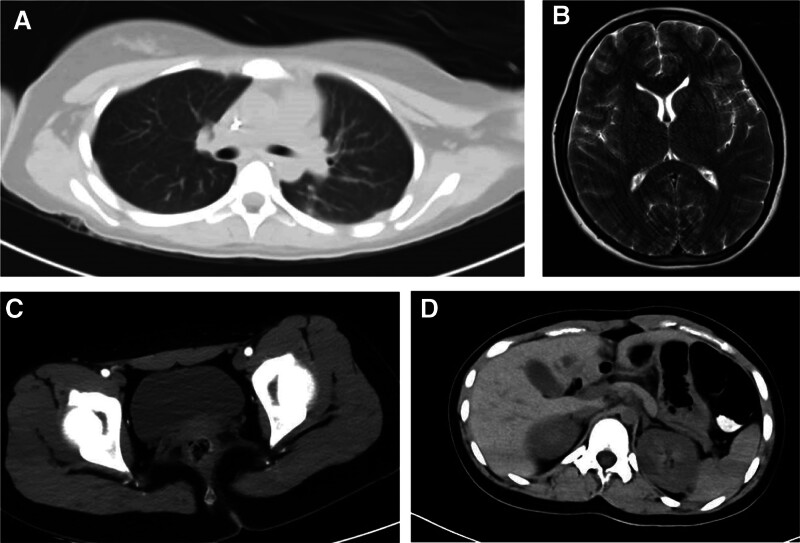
Imaging of the patient after admission to the hospital. All imaging tests found no suspicious tumors. (A) The result of the chest CT scan. (B) The result of the brain MRI. (C) The result of the pelvis CT scan. (D) The result of the abdomen CT scan.
